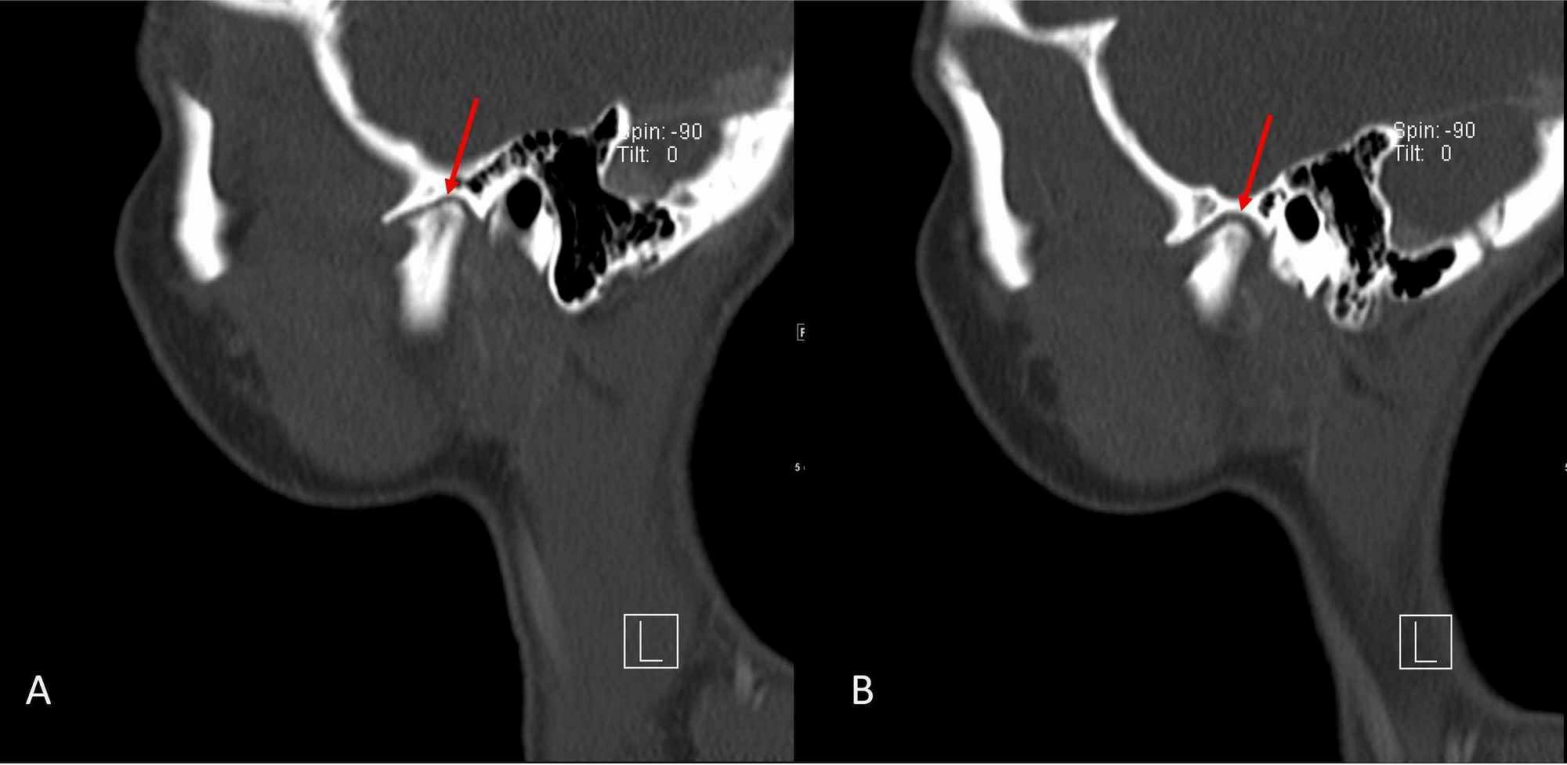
Sagittal non-contrast maxillofacial computed tomography (CT) (bone window) Left (A) and right (B) demonstrate resorption/flattening of the bilateral mandibular condyles with severe temporomandibular joint space narrowing.
